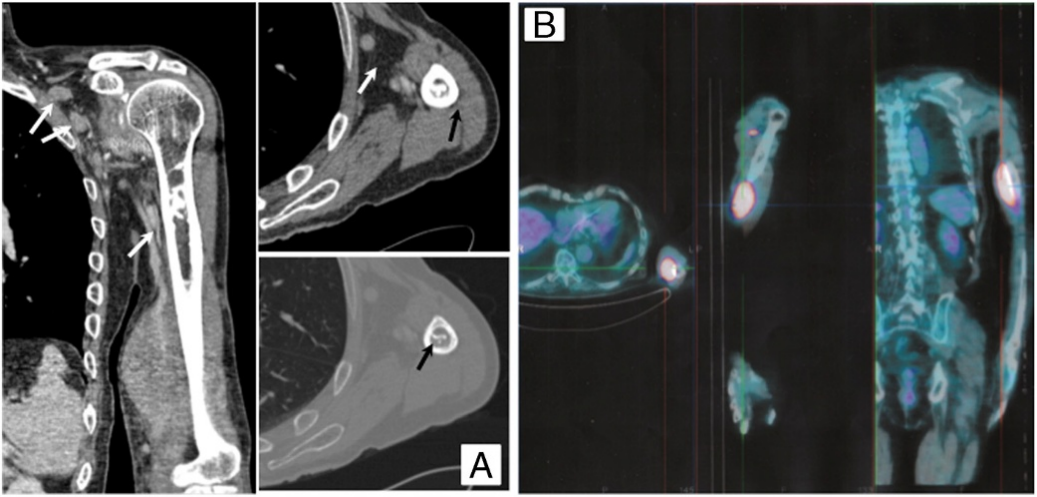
Computed tomography (CT) and Positron emission tomography (PET) images at the time of diagnosis. Panel A: CT scan showing the pathological tissue of the mid-diaphysis of the left humerus infiltrating the adjacent biceps brachii muscle for a longitudinal extent of 10.5 cm and axial dimensions of up to 5.5 cm. Several lymph nodes placed in the supraclavicular (1 cm), subclavian (2 cm) and in the axilla (19 mm). Panel B: PET image at the diagnosis with FDG uptake restricted to the primary mass, sparing locoregional lymph nodes.
